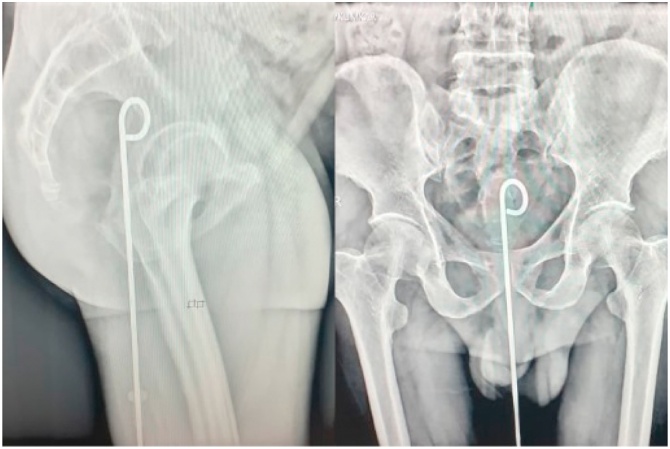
Represents the pelvic radiograph showing the metallic foreign body with crooked end.
